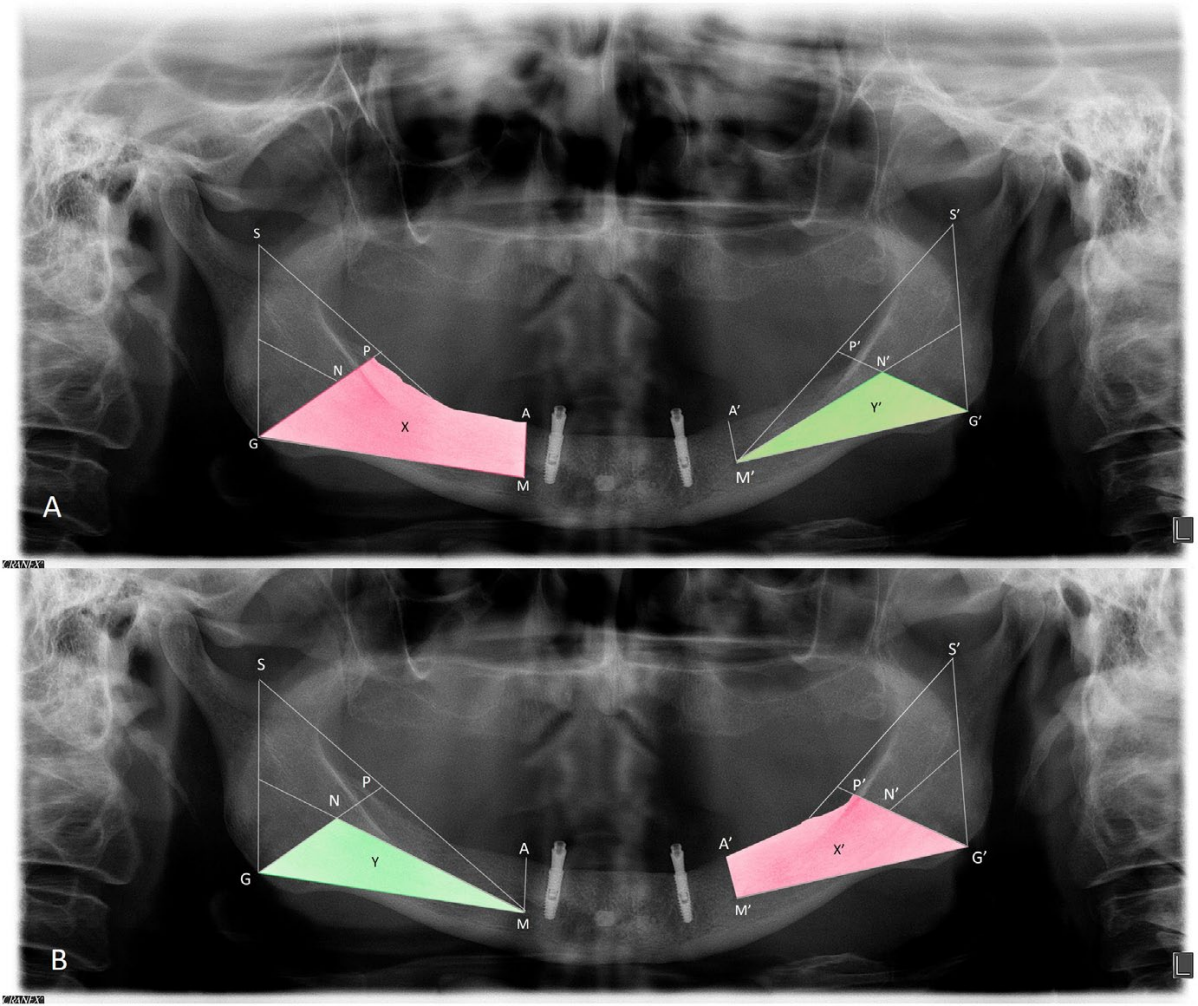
Demonstration of the areas for calculating the PAI in the mandible using panoramic radiographs to determine experimental and reference area regions: (A) right reference area (Y) and left experimental area (X); (B) right experimental area (X′) and left reference area (Y′).
